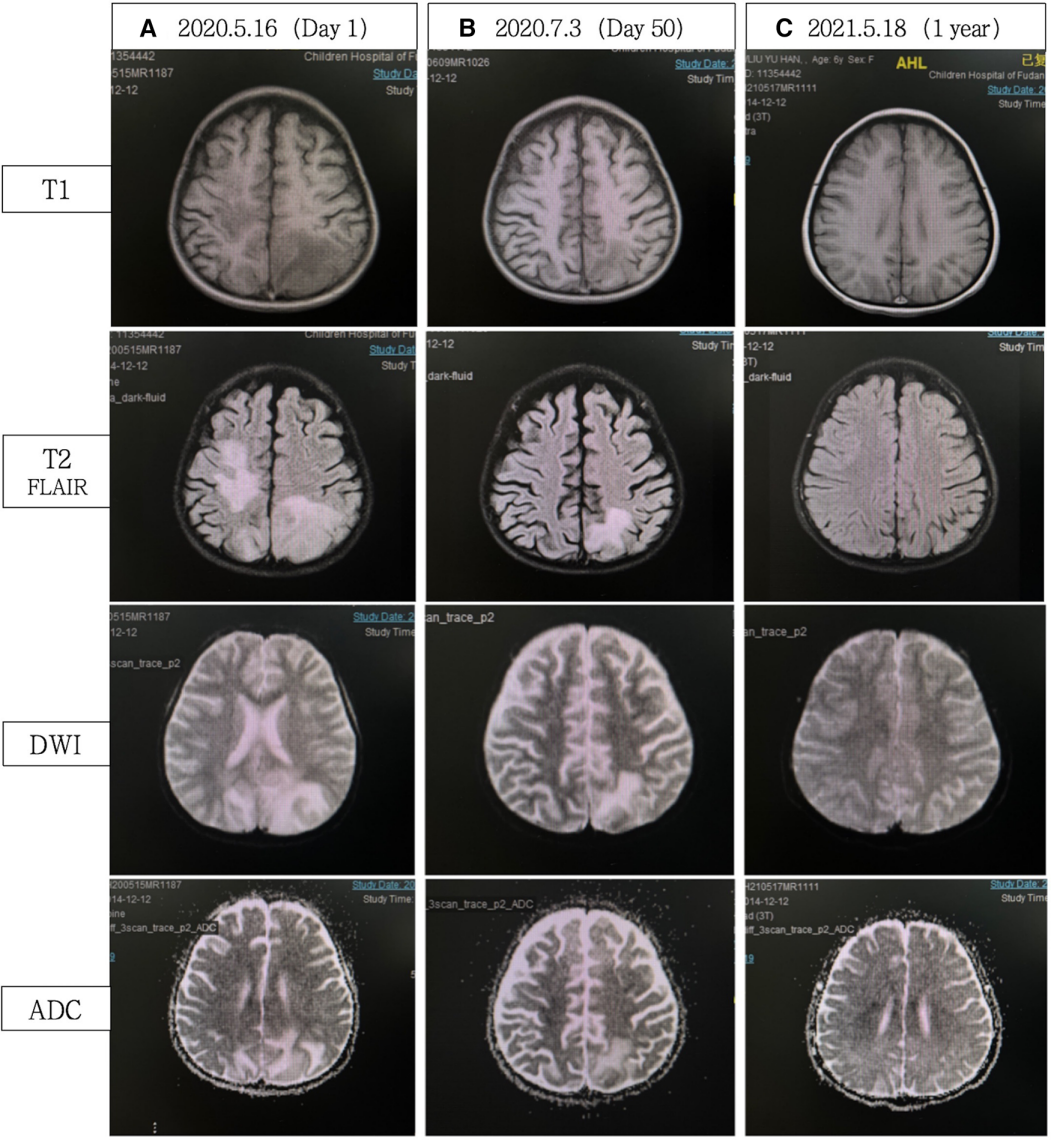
(A) Cerebral MRI demonstrated extensive bilateral T2 FLAIR/DWI hyperintense and T1 hypointense lesions, without ADC value reduction. (B) Significant reduction in abnormal signals compared with A. (C) Complete resolution of abnormal signals. FLAIR, fluid attenuated inversion recovery; DWI, diffusion weighted imaging; ADC, apparent diffusion coefficient.
